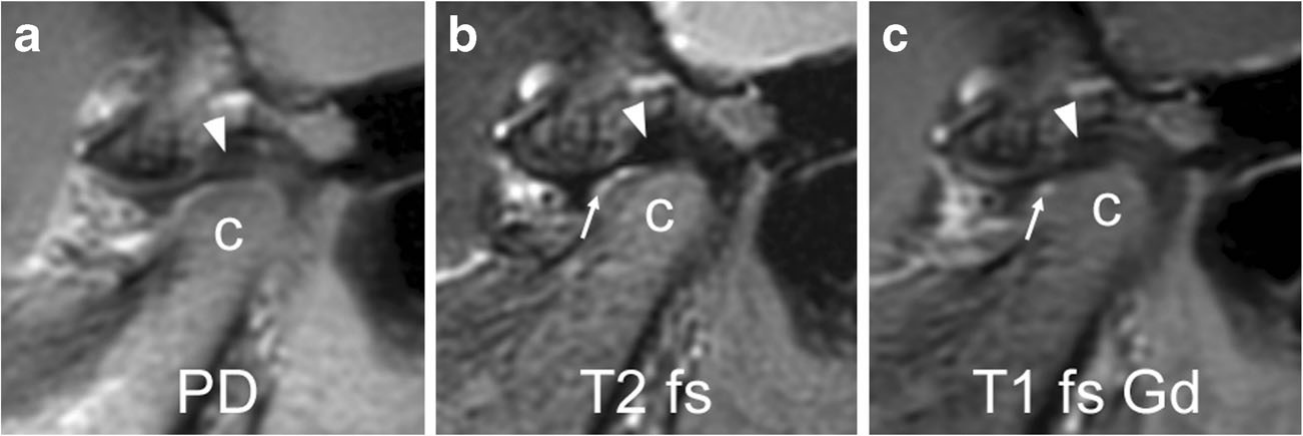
Normal articular disk in a 6-year-old girl. a-c Proton density weighted (TR/TE 3,000/13 ms, a), fat-saturated T2-weighted (TR/TE 5,400/77 ms, b), and postcontrast fat-saturated T1-weighted (TR/TE 670/10 ms, c) fast spin echo images all show a normal biconcave shape of the articular disk and the posterior band (arrowheads) located at the 11 o’clock position of the mandibular condyle (c). Note normal joint enhancement (arrow in c) confined to small amounts of fluid in the anterior lower joint space (arrow in b) and mild flattening of the anterior portion of condyle. PD proton density, T1 fs Gd contrast-enhanced fat saturated T1-weighted, T2 fs fat saturated T2-weighted
